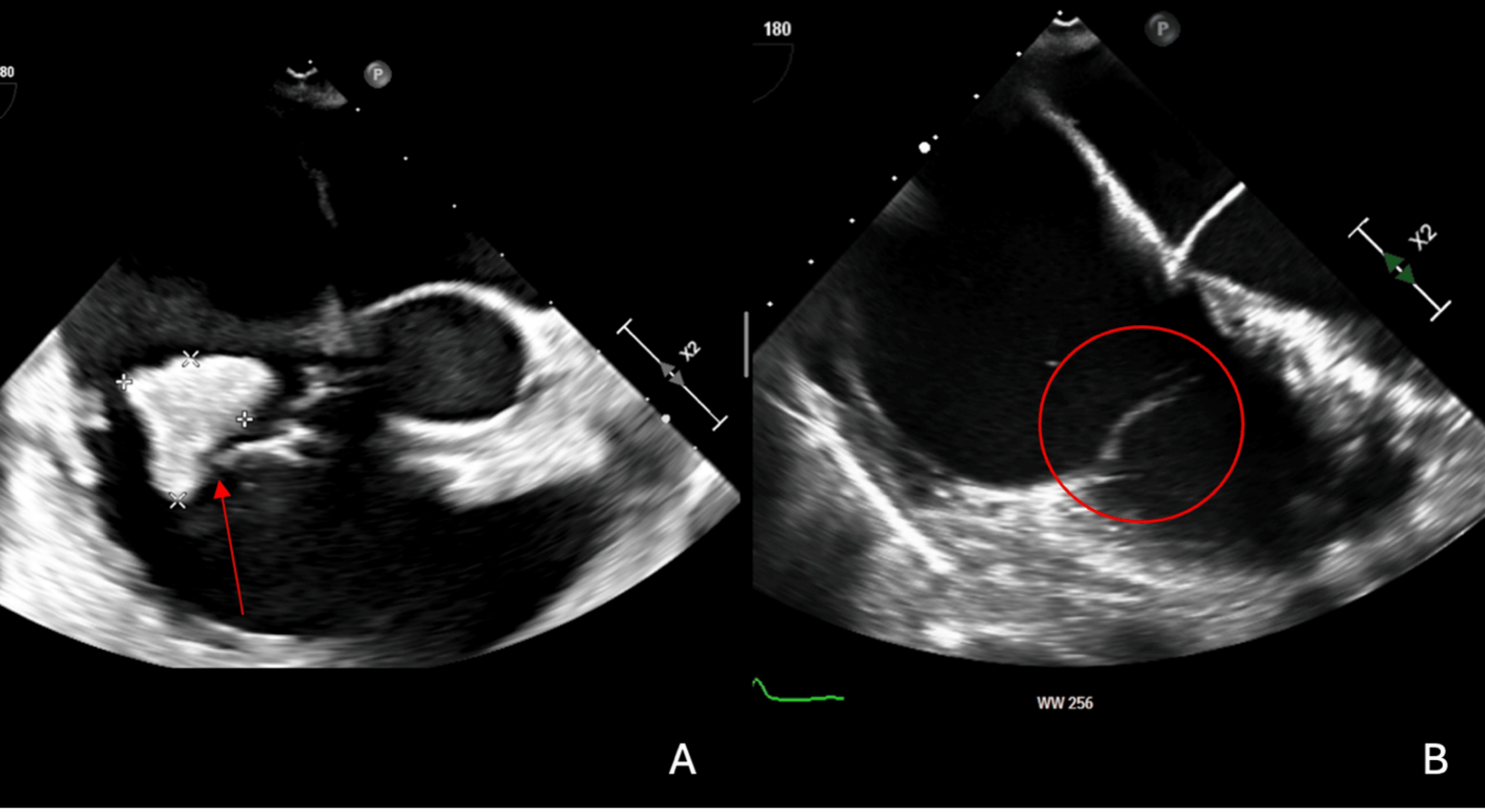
(A) TTE of the 2.09 cm x 1.8 cm large mobile vegetation between the anterior and posterior leaflets of the TV (red arrow). (B) TTE of the 0.63 cm x 0.49 cm on the tip of the posterior tricuspid leaflet on the atrium side (red circle). ‘A’ TTE from the initial visit; ‘B’ TTE from the second visit two months later TTE: transthoracic echocardiogram, TV: tricuspid valve
